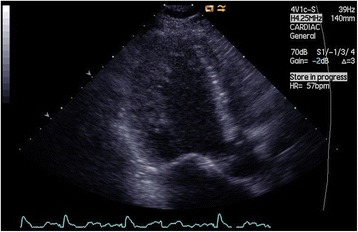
Transthoracic echocardiogram, systole, apical long-axis view showing a thick non-compacted myocardium in the left ventricular wall
